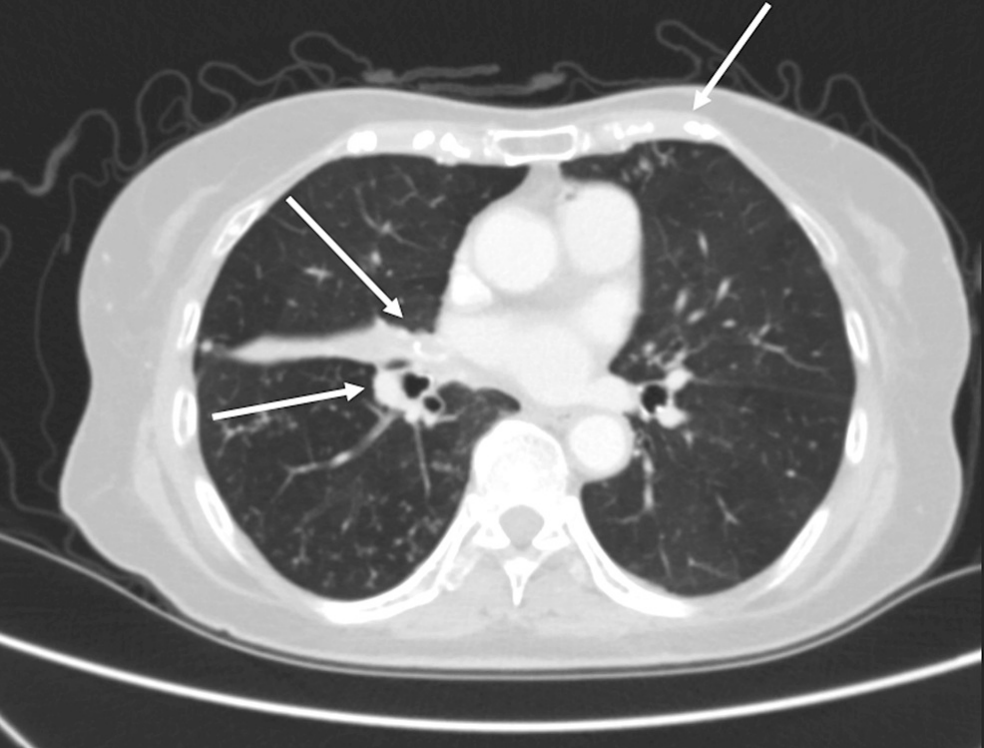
CT chest showing calcified mediastinal adenopathy, nodular pleural thickening, and bronchus obstruction in the right lung lobe
